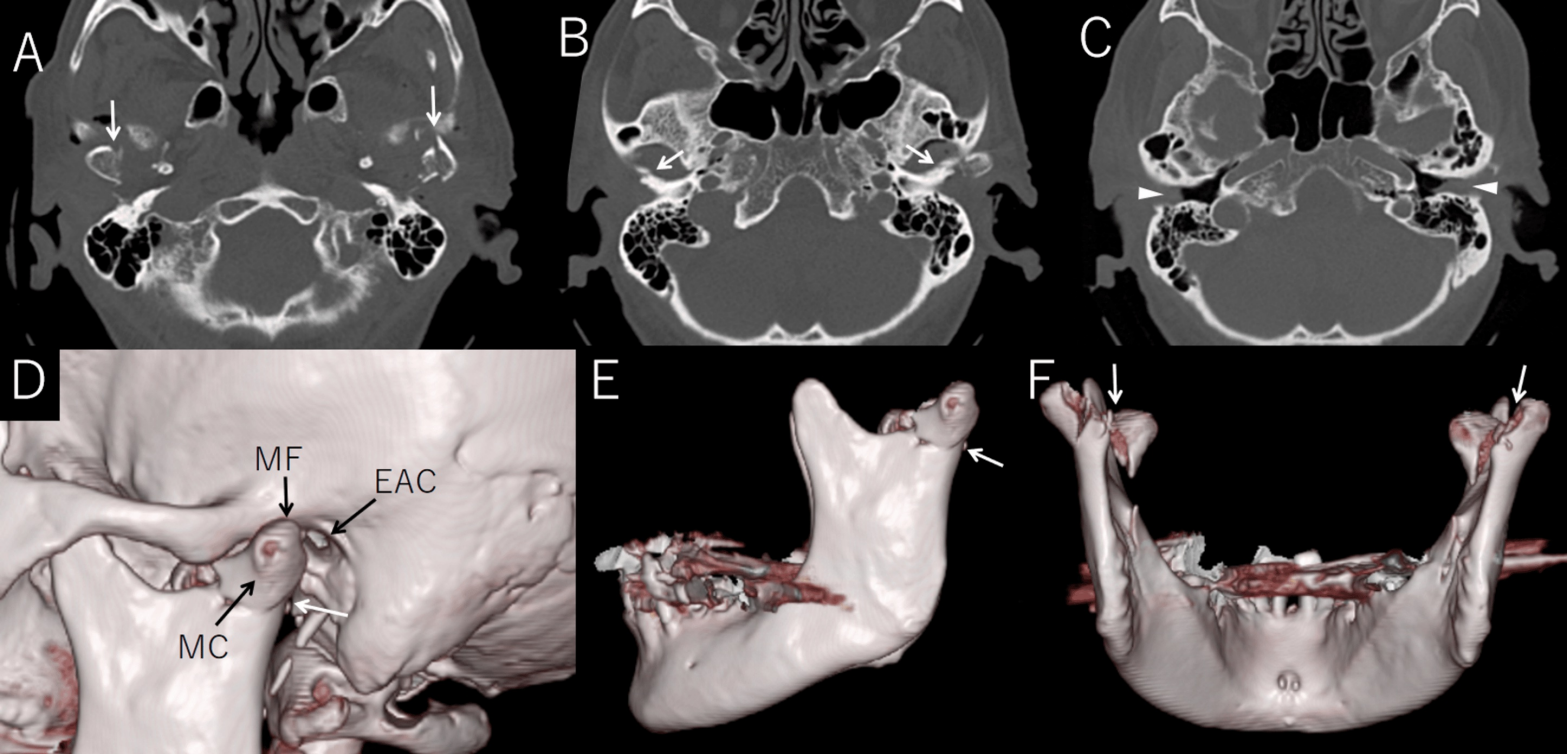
CT and 3D reconstruction images demonstrating bilateral mandibular condyle fractures and associated external auditory canal injury Axial CT images (A–C) show bilateral mandibular condyle fractures (white arrows in A), a fracture of the anterior wall of the left external auditory canal (white arrows in B), and soft tissue density in both external auditory canals indicating hematoma (arrowheads in C). Three-dimensional CT reconstructions (D–F) reveal the anatomical relationship between the mandibular condyle (MC), mandibular fossa (MF), and external auditory canal (EAC) (D), as well as bilateral condylar fractures clearly visible from lateral (E) and posterior (F) views (white arrows).
